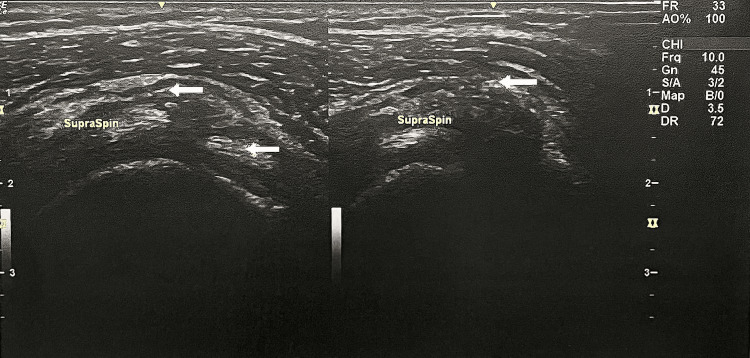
Ultrasound image of calcific deposit, shown by arrows.
